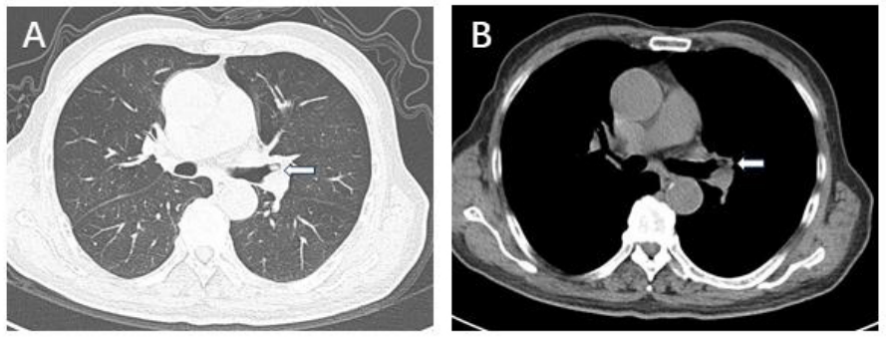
Medical image. A: Chest CT scan (lung window) shows a round-like nodule within the lumen of the left upper lobe bronchus (arrow). B: Mediastinal window shows the nodule within the left upper lobe bronchus (arrow).
